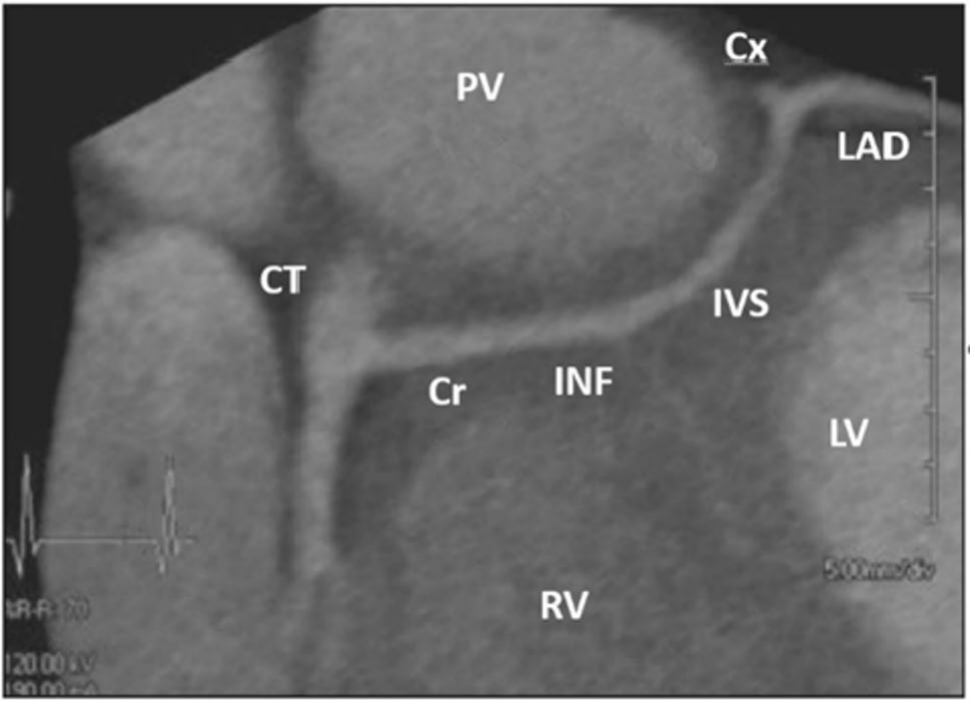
Typical case of L-ACAOS-IS focusing on the intramyocardial segment of the left main artery. An extramural common trunk (CT) comprises the right coronary artery and the initial segment of the left coronary artery, which branches at the unusually low-splitting cristal level (Cr) before passing through the infundibular segment and upper septum (IVS) [22]. The LAD is epicardial. *CT* indicates common trunk, *Cr* crista supraventricularis, *Cx* circumflex coronary artery, *INF* infundibular segment, *IVS* interventricular septal segment, *L-ACAOS-IS* anomalous origin of the left coronary artery from the right sinus of Valsalva, with intraseptal course, *LAD* left anterior descending coronary artery, *LV* left ventricle, *PV* pulmonary valve, *RV* right ventricle. Adapted from Nath H, Singh SP, Lloyd SG. CT distinction of interarterial and intraseptal courses of anomalous left coronary artery arising from inappropriate aortic sinus. *AJR Am J Roentgenol.* 2010;194(4):W351-352
